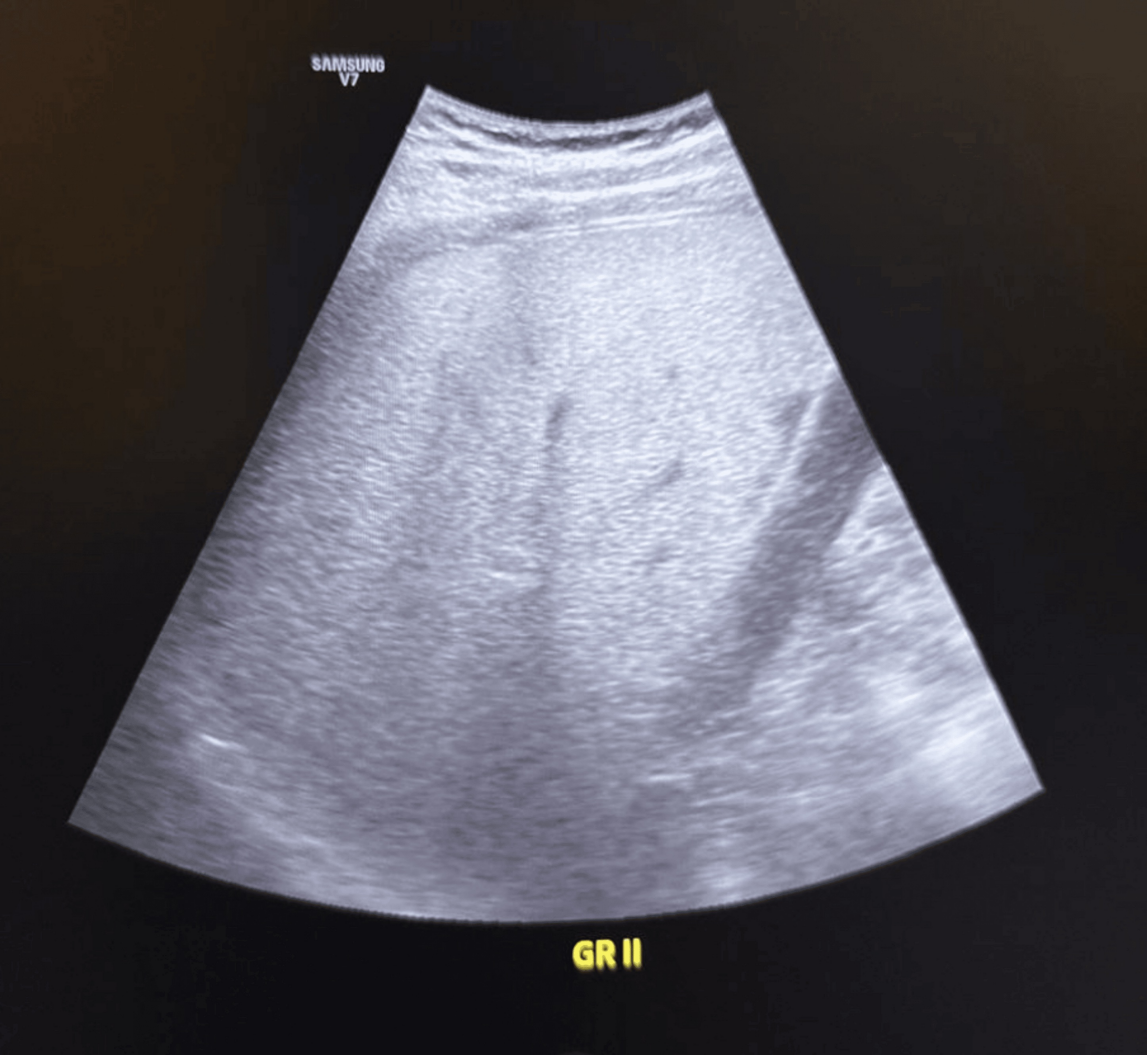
Abdominal ultrasound findings
